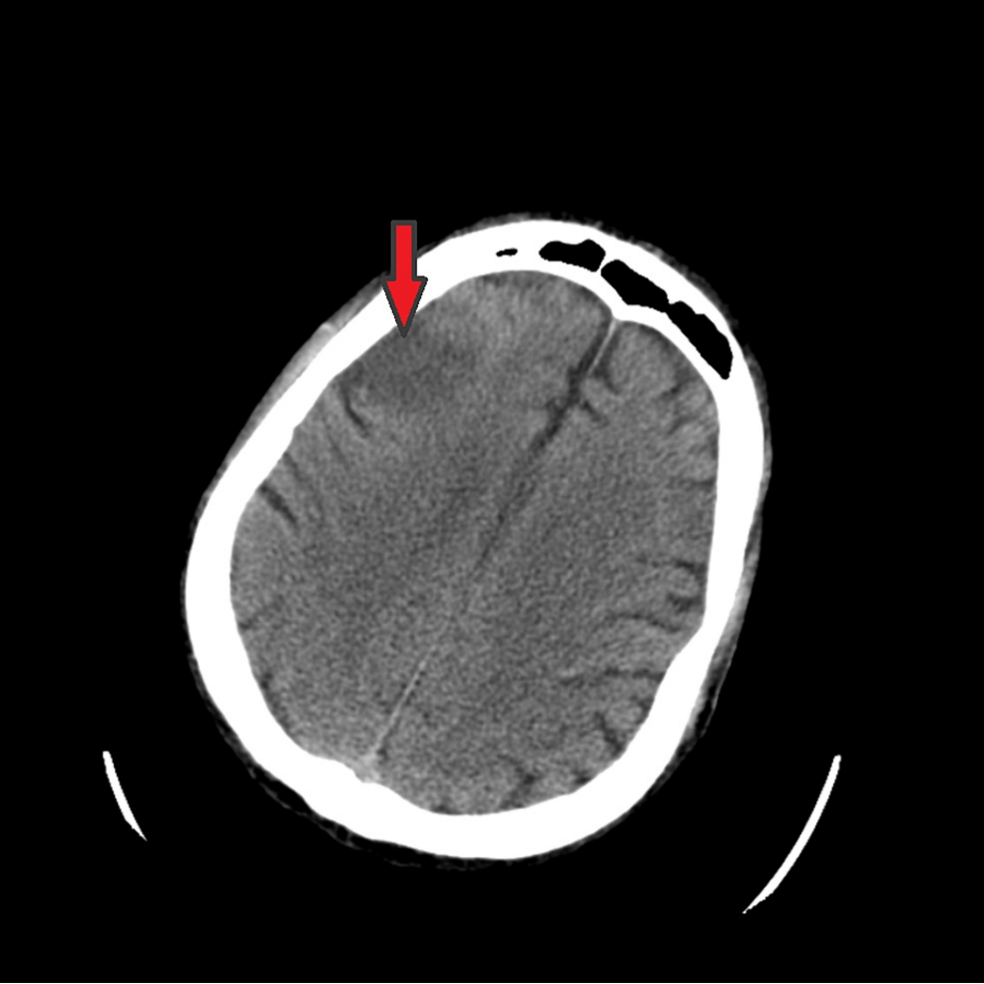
CT scan of the head demonstrating right frontal cytotoxic edema
